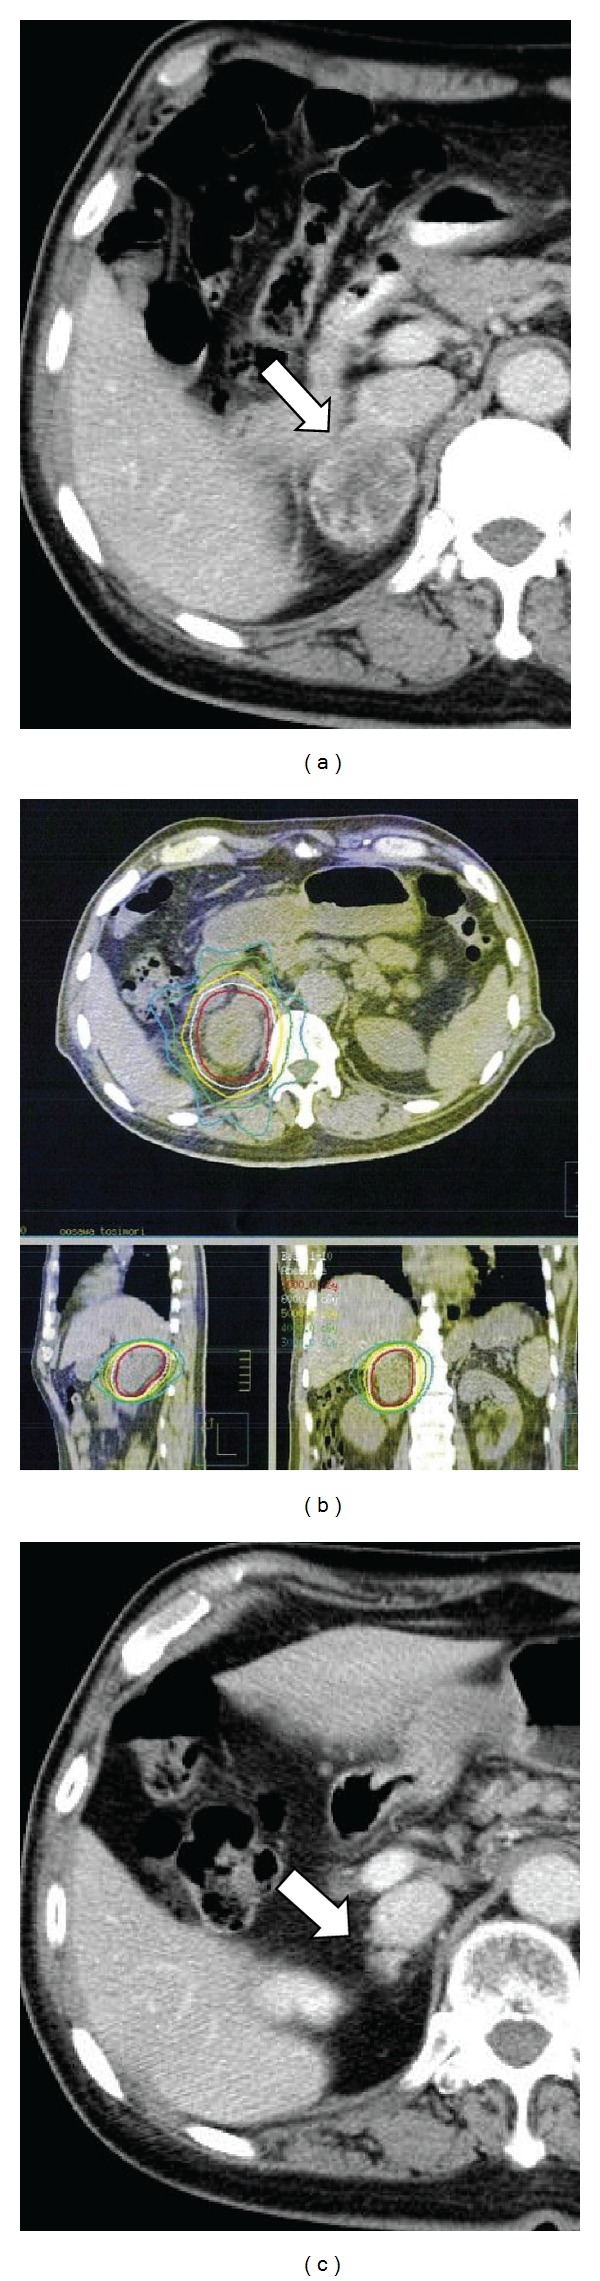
(a) CT of right adrenal metastasis (arrow) before SBRT. (b) Dose distribution made with 10 noncoplanar beams for SBRT. Isodose lines show total doses (in 25 fraction) of 70 Gy, 60 Gy, 50 Gy, and 40 Gy, in 10 fractions, respectively, from the innermost area. The 30-Gy isodose line overlapped at the second portion of the duodenum with the 40-Gy isodose line of the SBRT for right adrenal metastasis, resulting in grade 2 duodenitis 1 month after SBRT. (c) CT at 6 months after SBRT, showing partial response of the lesion (arrow).
